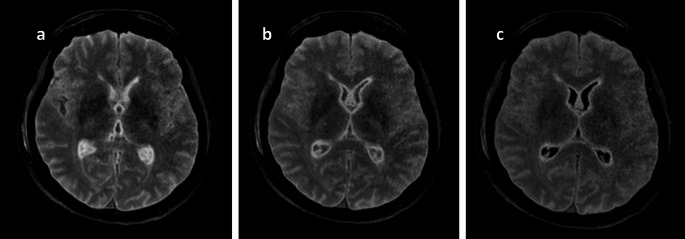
Example of FLAIR axial reconstructions of the brain for a case at 16,1 °C: a) TI 1660 ms (original); b: TI 1440 ms; c: TI 1220 ms, in which the image c) was considered as a living-patient like contrast, with the CSF signal suppressed (appearing black on the image)
